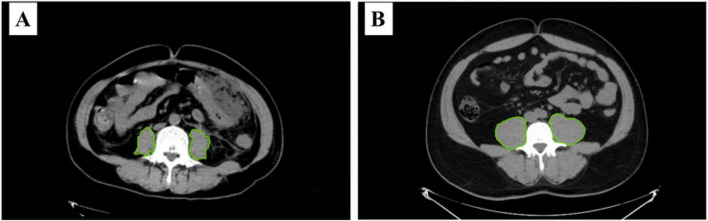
PMA measured on CT images of different patients. Representative axial CT images showing the psoas muscle (outlined in green) at the level of the third lumbar vertebra from a patient with low PMA (A) and a patient with high PMA (B).
